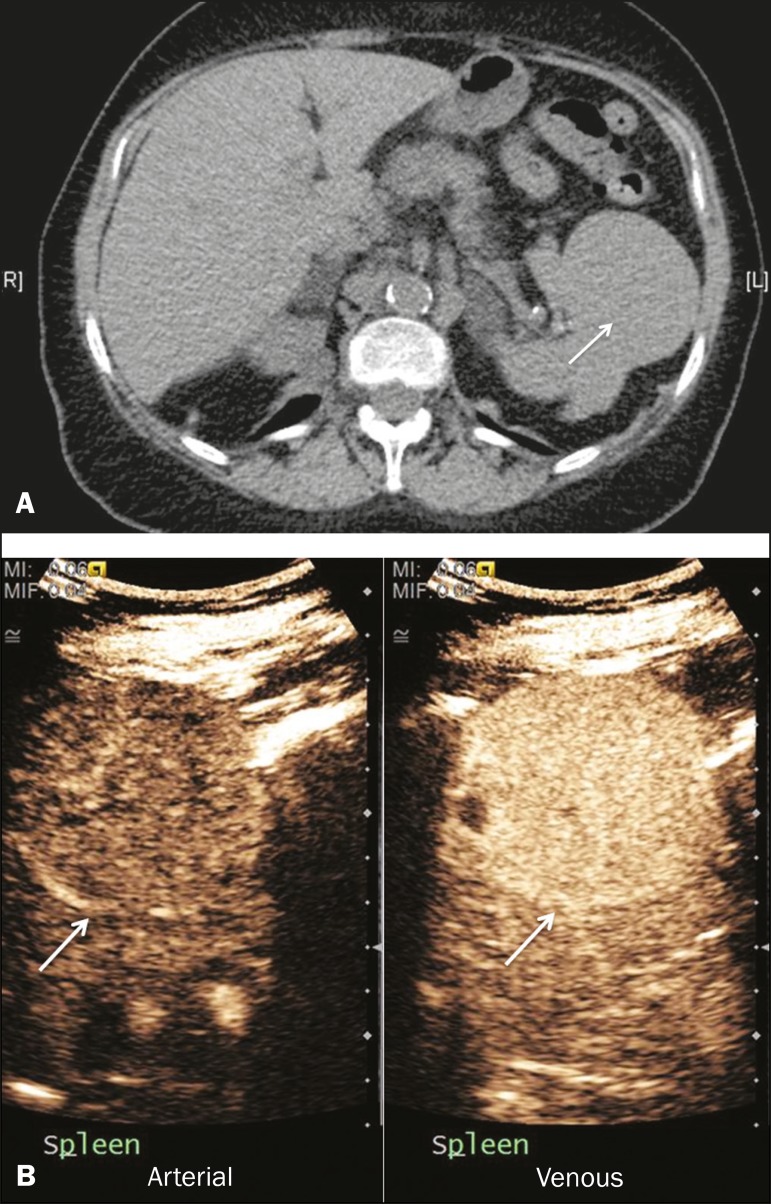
Splenunculi. A: Non-contrast-enhanced CT scan, performed
